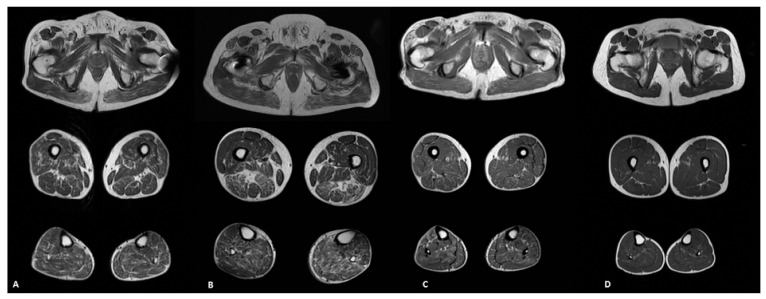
Muscle MRI pattern of fatty infiltration in ATTRv patients (#2 in (A); #6 in (B); #1 in (C)) and in a healthy subject (D). Tensor fascia latae and soleus were the most frequently involved muscles, with relative sparing of the iliopsoas, rectus femoris, gracilis and anterior tibilias muscles (A,B); in only one case the anterior tibialis presented an asymmetric severe adipose substitution (C). No fatty replacement was found in healthy subject (D).
